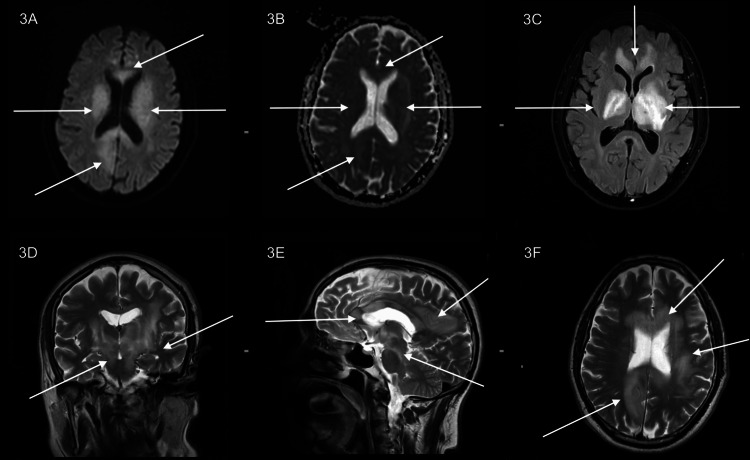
(A, B) DWI and ADC images showing diffusion restriction in bilateral gangliocapsular regions, periventricular white matter and corpus callosum. (C) Axial FLAIR images showing hyperintense signal intensity in bilateral gangliocapsular regions, periventricular white matter and corpus callosum. (D, E, F) T2 coronal, T2 sagittal and T2 axial: Multiple lesions showing heterogeneous signal intensity with hyperintense oedema involving corpus callosum, gangliocapsular regions, bilateral periventricular white matter, brainstem and cerebral peduncles. Compared to the study, as seen in Figures 1 and 2. Diffusion restriction is significantly more pronounced. There was an interval increase in the extent and size of the lesions. DWI: diffusion-weighted imaging; ADC: apparent diffusion coefficient; FLAIR: fluid-attenuated inversion recovery.
